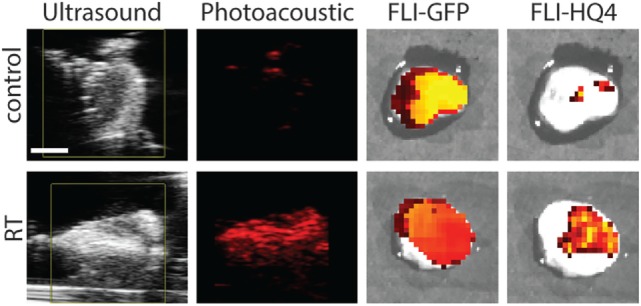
Ex vivo* photoacoustic and fluorescence imaging. Representative ultrasound, photoacoustic, GFP fluorescence (FLI-GFP) and HQ4-DTPA fluorescence (FLI-HQ4) images of control and irradiated tumors resected 40 h after injection of HQ4. Scale bar = 2 mm.
